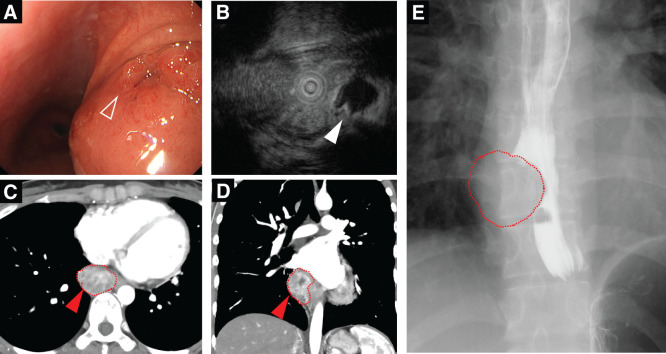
(A) Upper gastrointestinal endoscopy shows a submucosal tumor with a small papillary protrusion in the Lt (open arrowhead), covered by heterotopic gastric-type mucosa. (B) Endoscopic ultrasonography demonstrates a well-circumscribed, homogeneous hypoechoic mass (arrowhead) arising from the esophageal wall. (C, D) Contrast-enhanced CT (axial view in C; coronal view in D) reveals a well-defined extraluminal mass along the right wall of the Lt (red arrowheads). The dotted lines outline the extent of the lesion, which shows heterogeneous enhancement with focal internal low-attenuation areas. (E) Barium esophagography demonstrates a smooth-contoured elevated lesion along the right wall of the Lt (dotted outline). Lt, lower thoracic esophagus
